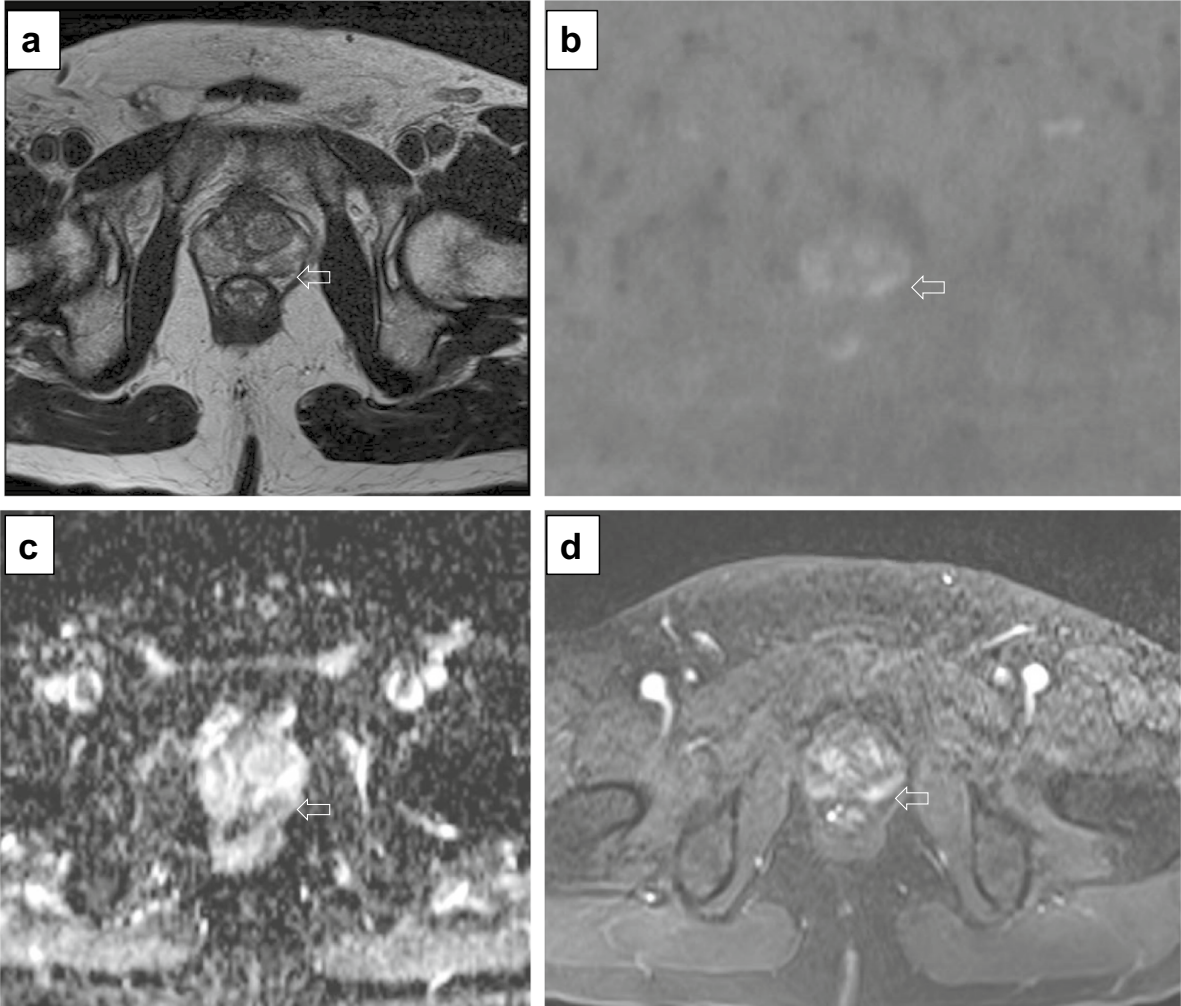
Axial T2w imaging (a), high *b* value (b), ADC map (c) and DCE acquisition (d) of a study that was given a PI-QUAL score of 2. Only T2w imaging (a) is of acceptable diagnostic quality (although the slice thickness is 3.5 mm). The in-plane resolution (including the ADC map) and the slice thickness (5 mm) of DWI and the in-plane resolution and slice thickness (3.5 mm) of DCE sequences are below the minimum standard of diagnostic quality as per PI-RADS v.2.1 technical recommendations. The arrows indicate a lesion in the left peripheral zone between 5 and 6 o’clock. Targeted biopsy revealed Gleason 3 + 4 disease
